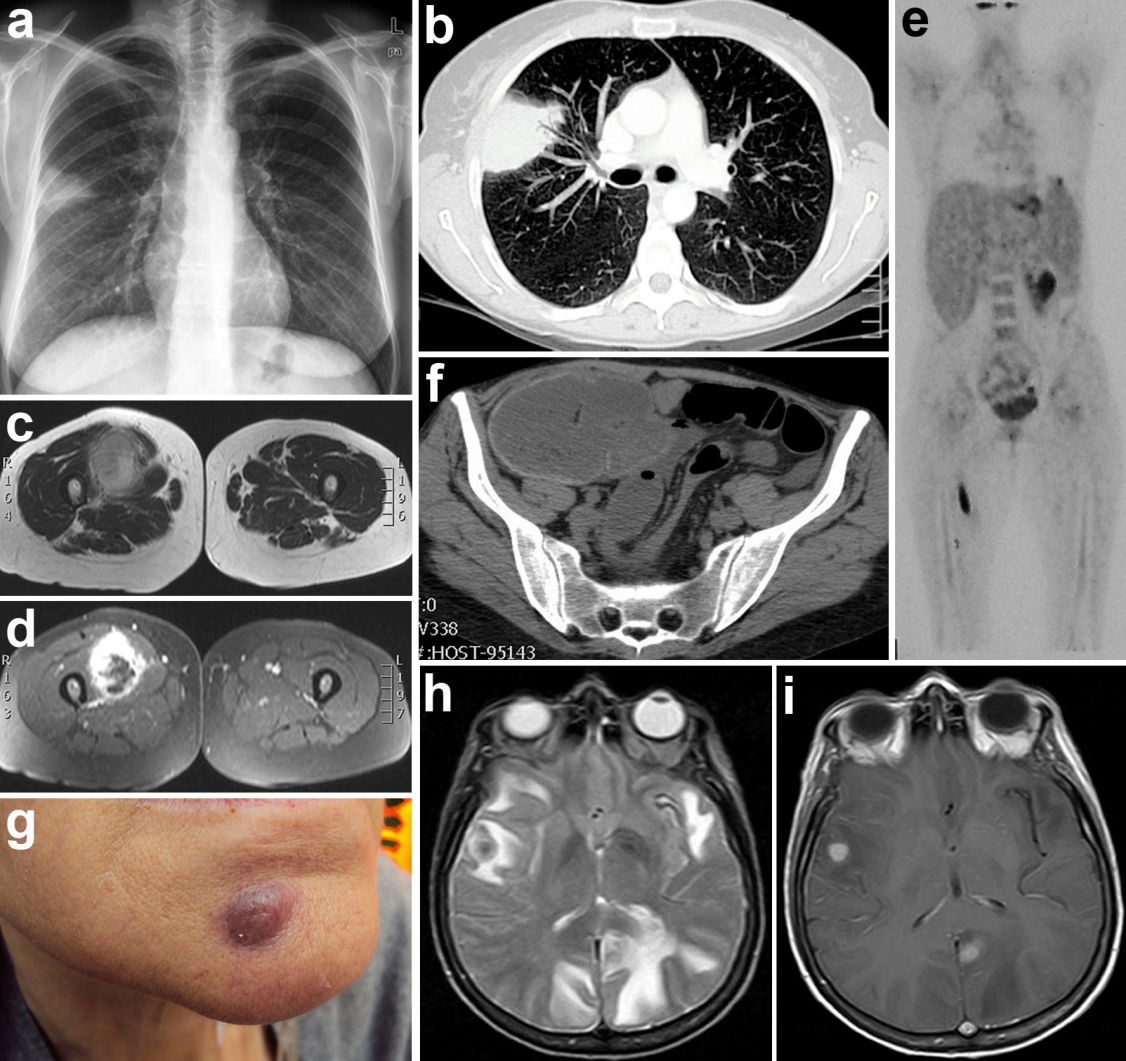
R. equi *lesions in disseminated infection (case report 2). a Chest radiograph (May 2005) shows a non specific subpleural opacity in the right upper lobe without evidence of pleural effusion. b Contrast enhanced multidetector computed tomography (MDCT)(May 2005). A scan at the level of the main bronchi demonstrates a subpleural focal consolidation in the right upper lobe. There is no evidence of lymphoadenopathy or pleural effusion. c, d Contrast enhanced magnetic resonance imaging (MRI) of the right thigh (August 2009). The axial T2 sequence (c) and the axial T1 contrast enhanced sequence with fat suppression (ccGRE T1FS) (d) show an oval shaped enhancing mass in the vastus medialis muscle with central area of necrosis and oedema of the surrounding tissue and muscle. e Positron emission tomography (PET) and computed tomography (CT) images (August 2009) show focal increased fluodeoxyglucose (FDG) uptake in the upper lobe of the right lung and in the spleen, which is larger than normal, a large area of increased uptake in the right lower abdomen consistent with colon localization, and an irregular area of increased FDG uptake in the soft tissue of the right proximal thigh. f MDCT of the abdomen (March 2010). A scan through the lower abdomen shows a large obstructing mass in the right colon with stranding of the pericolonic fat and several enlarged lymph nodes. g Cutaneous nodular rhodococcal lesions (March 2010). h Brain axial T2 weighted sequence (August 2010) shows multiple (right temporal, left mesial occipital, left temporoinsular) expansive oedematous lesions. All the lesions show central hypointensity and peripheral hyperintensity. Oedema is also present in right occipital and anterior temporal lobes. i Brain axial T1 weighted sequence after Gd injection (August 2010) shows enhancement of the two nodular lesions in right temporal region and in left occipitomesial lobe. Smooth cortical enhancement is also seen in the left occipital lobe.
